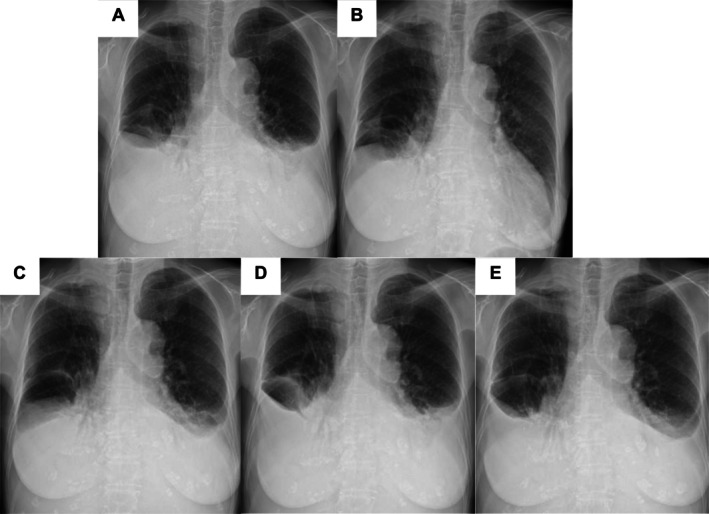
Serial chest radiographs showing the progression of bilateral pleural effusions at five time points. (A) Baseline chest radiograph showing bilateral pleural effusions before intervention. (B) Radiograph after left‐sided pleural drainage showing decreased effusion on the left, whereas the unpunctured right effusion shows a slight decrease. (C) Increased pleural effusion exclusively on the left side without further intervention. (D) Following equilibration of effusion volumes between both sides, a subsequent bilateral increase was observed. These sequential changes shown in (A–D) may reflect redistribution of pleural fluid influenced by intrathoracic pressure dynamics and are consistent with pleural fluid accumulation secondary to retrograde lymphatic flow. (E) Radiograph obtained after initiation of a fat‐restricted diet showing no further increase in effusion volumes, indicating stabilisation.
